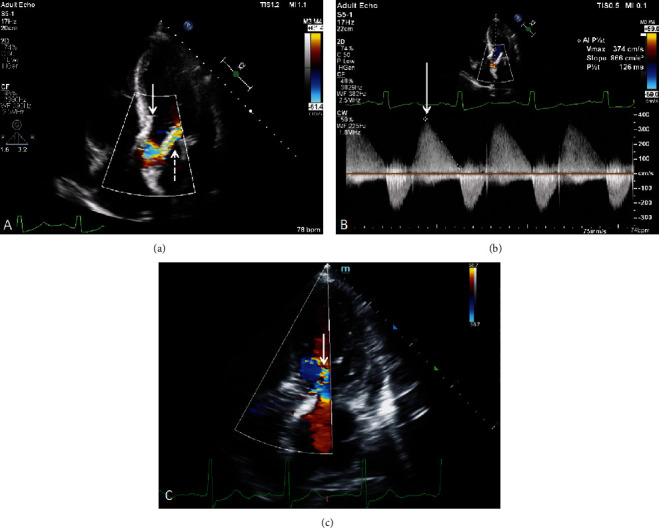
Transthoracic echocardiography. (a) Color Doppler showing left ventricular migration of an aortic bioprosthesis (solid arrow) resulting in PVL (dashed arrow). (b) Spectral Doppler showing a rapidly decelerating (triangle-shaped) aortic regurgitation jet (solid arrow) with a pressure half − time = 126 msec suggestive of severe PVL. (c) Transthoracic echocardiogram 24 hours post-TAVR for comparison. It demonstrates a higher valve position and only mild to moderate PVL when compared to (a).
